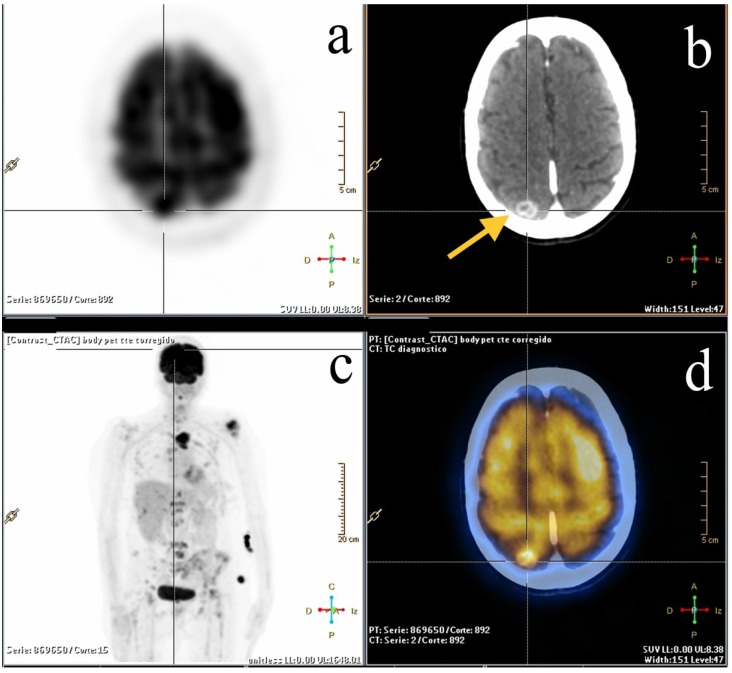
A 67-year-old woman with breast carcinoma and known bone metastases, but without suspicion of progression, referred for FDG-PET/CT exam. (a) Axial PET image showing a focus of FDG uptake in the right posterior parietal lobe; (b) axial CT showing a rounded lesion with contrast enhancement ring and perilesional edema (arrow); (c) MIP image demonstrating the known multiple bone metastases; (d) fused image showing a rounded hypermetabolic new lesion consistent with brain metastases.
